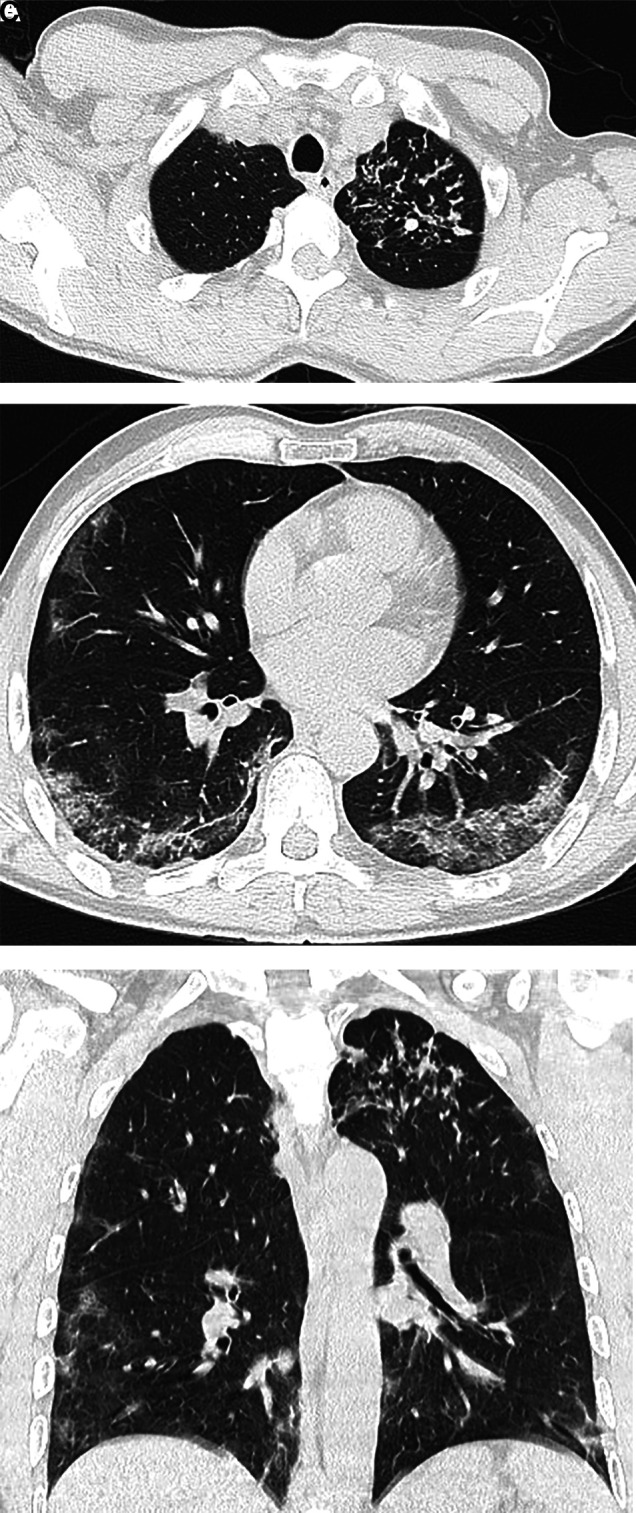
(A) Tree-in-bud signs in the left upper lobe. (B) Bilateral ground-glass opacities in a peripheral distribution. (C) Coronal computed tomographic scan shows both tree-in-bud pattern and peripheral ground-glass opacities.
